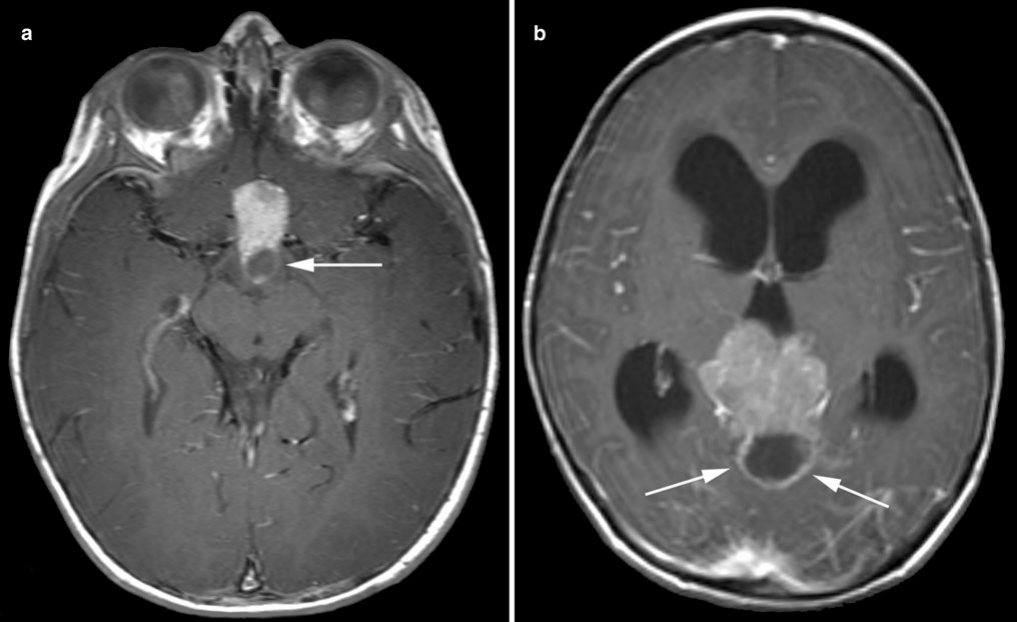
Suprasellar and pineal gland trilateral retinoblastoma. Contrast-enhanced axial T1-weighted images showing solid tumor masses with cystic components in both the suprasellar region (patient 15) (a) and pineal gland (patient 7) (b). The pineal gland mass causes a secondary hydrocephalus because of brainstem compression (b)
